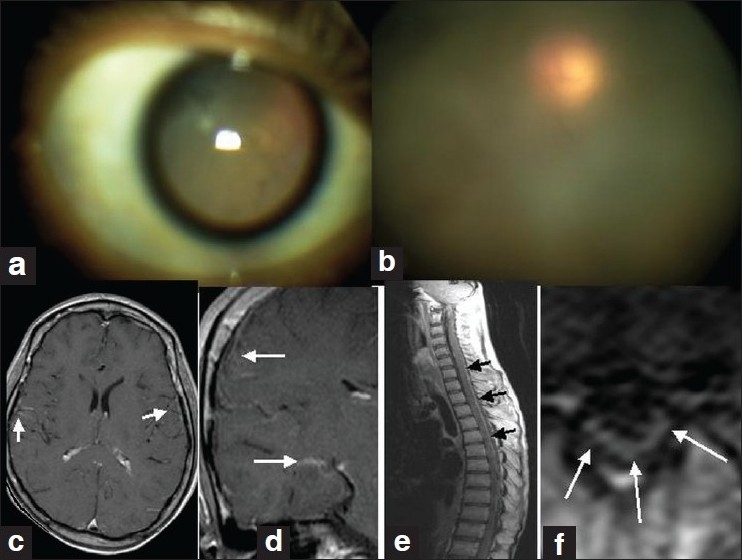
a–e: (a) Dilated pupil; (b) hazy vitreous; (c and d) postcontrast axial and coronal T1W MRI of brain showing leptomeningeal enhancements (arrows); (e and f) post-contrast sagittal and axial T1W MRI of spine with extensive meningeal enhancement
